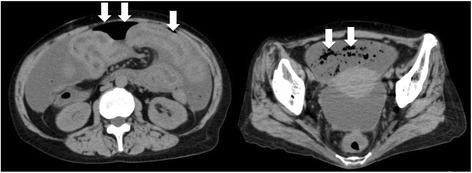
Ascites and abdominal free air (arrows) were shown by abdominal computed tomography
